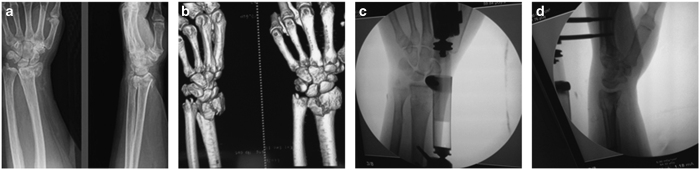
Radiographs of a 68-year-old man with a displaced intra-articular fracture of the distal radius that was treated by external fixation. (a and b) Anteroposterior and lateral radiograph before operation; (c and d) Anteroposterior and lateral radiograph during operation.
